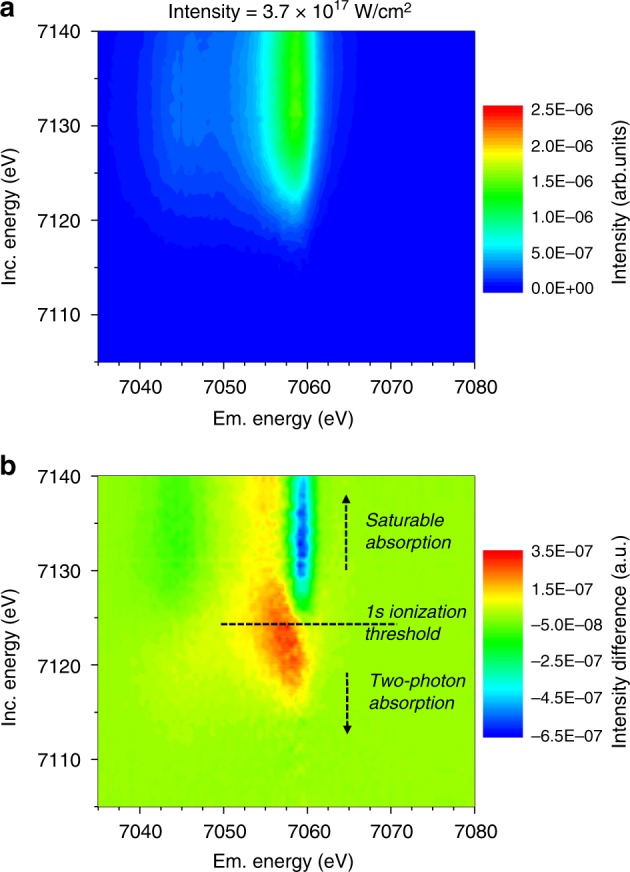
Reconstructed RXES maps for different incident intensities. The reconstructed RXES maps for the highest average X-ray intensity of the SASE pulses on the sample a and the difference map b towards the measurement at the lowest intensity, that is the RXES map from Fig. 2, c, are shown. In the vicinity of the core shell ionization threshold noticeable changes, with relative intensity differences of up to +17% and −32% can be observed. Notably a shift in the *1s* ionization threshold and a change in the intensity ratio between the Kβ1,3 and the Kβ′ emission lines, representative for the 3p-3d spin-coupling splitting, can be observed
